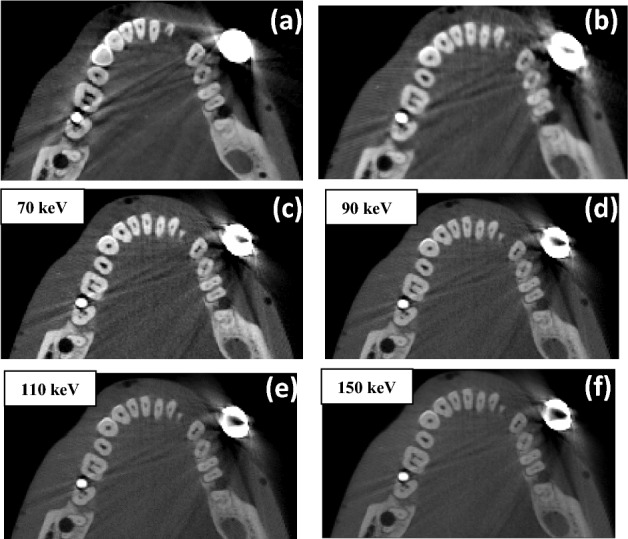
RANDO acquired with a clinical CBCT (a), the benchtop CBCT-1 (b) at 110 kV. (c,d,e,f) are VMI synthesized from the DE-CBCT dataset at 70, 90, 110 and 150 keV, respectively. Images are displayed with the same window of [-750 HU, 2250 HU].
